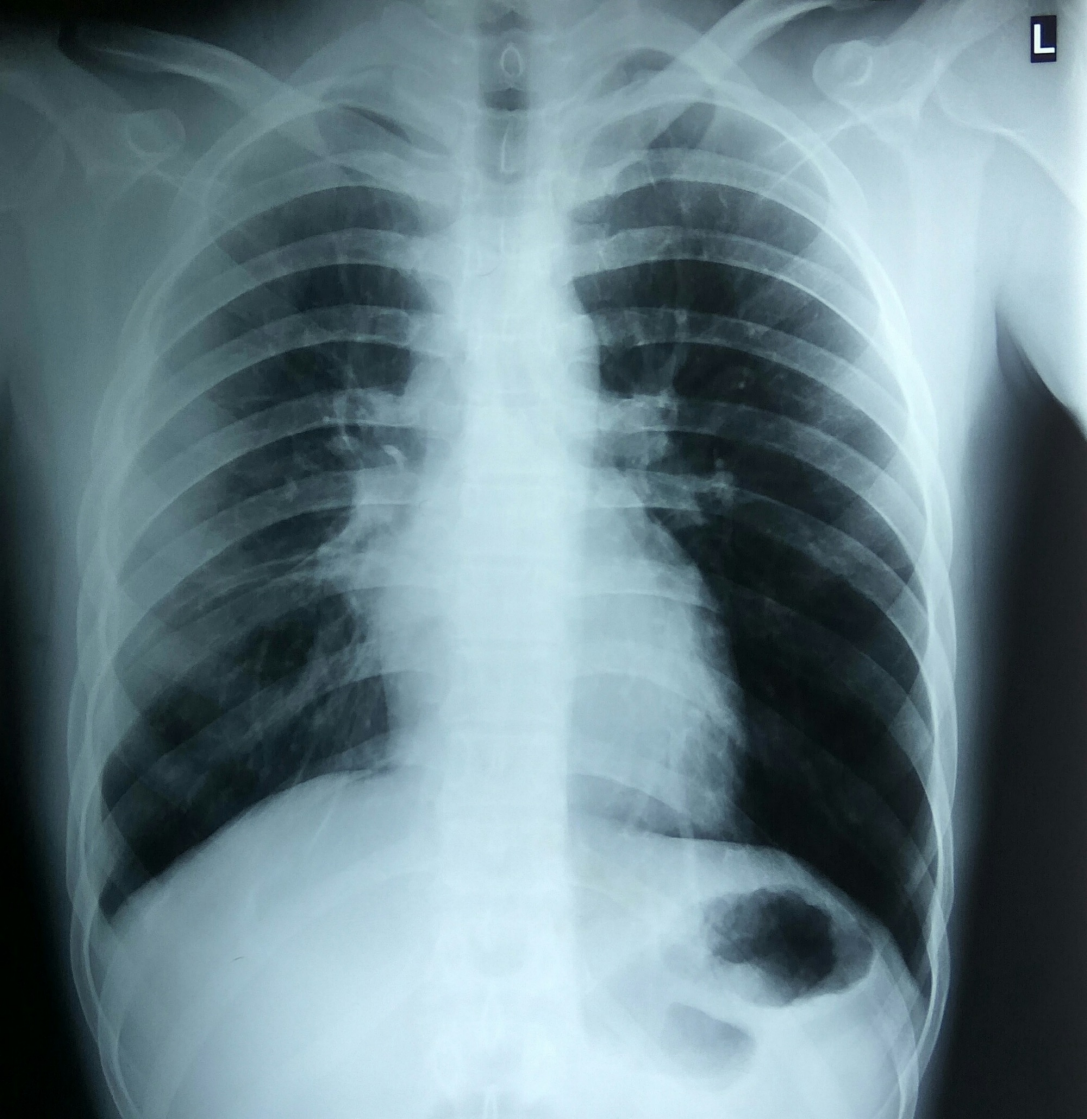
Posteroanterior erect chest X-ray of patient after one week of corticosteroid therapy.
